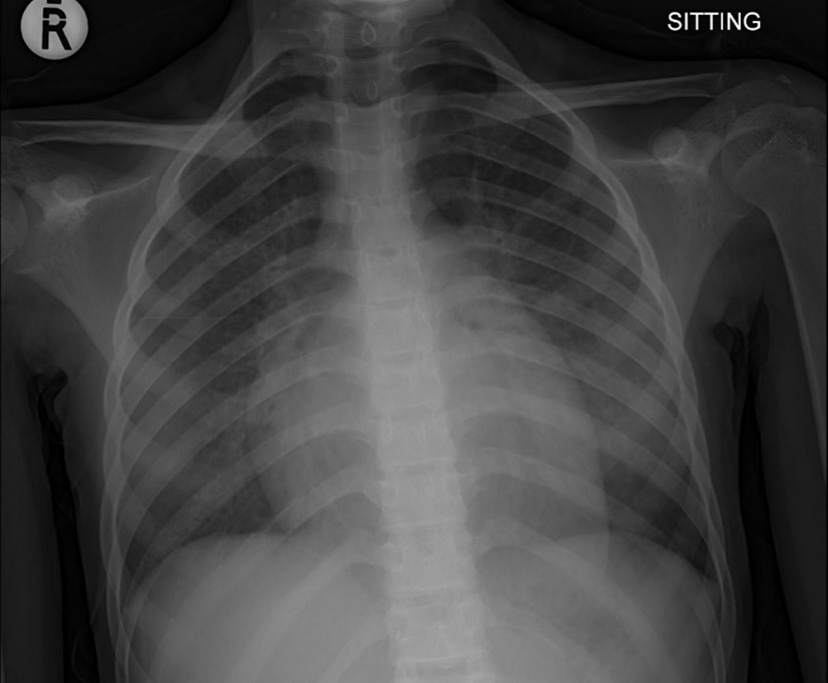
Preoperative chest x-ray showing cardiomegaly and signs of pulmonary edema. Note the splaying of the carina from the enlarged left atrium
